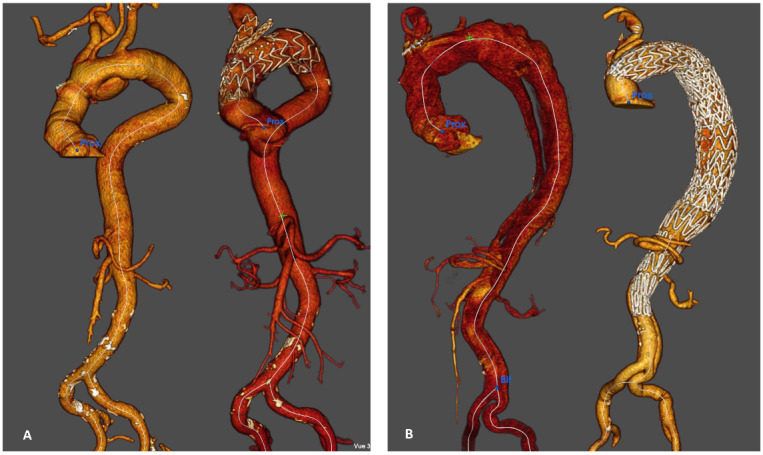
Branched endograft and hybrid repair for aneurysmal evolution of residual aortic dissection. (A): pre and post operative CT scan (volume rendering) after branched endograft for aneurysmal evolution limited to the aortic arch. (B): pre and post operative CT scan (volume rendering) after hybrid repair associated with TEVAR and STABILISE technique for a thoracic aneurysmal evolution.
